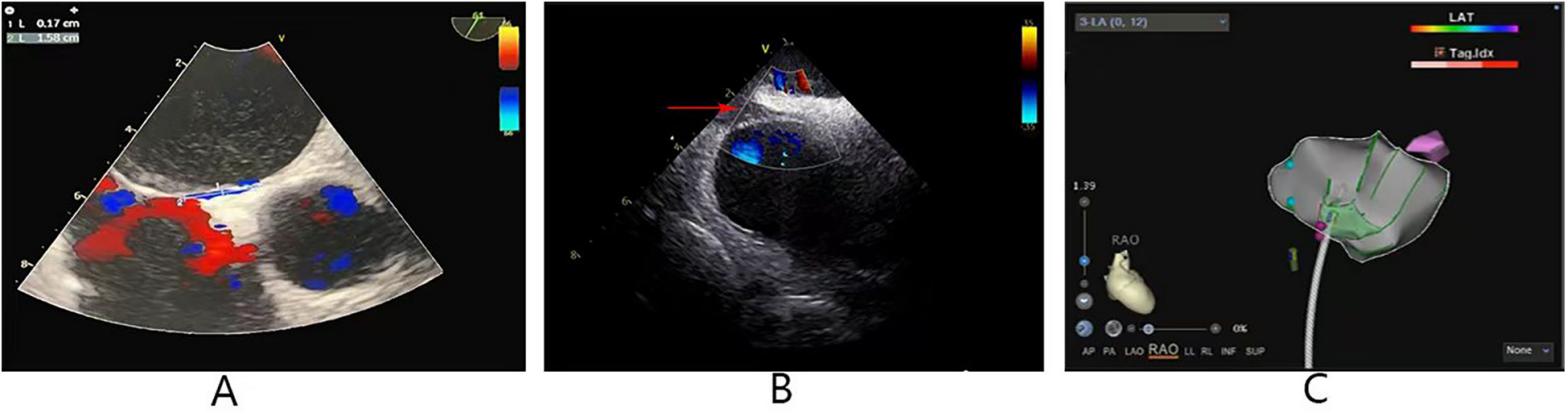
(A) Transesophageal echocardiogram showing a PFO: length 15.8 mm, width 1.7 mm. (B) The red arrow indicates that ICE view of PFO. (C) The Pink dots indicate PFO locations. PFO, patent foramen ovale; ICE, intracardiac echocardiography.
